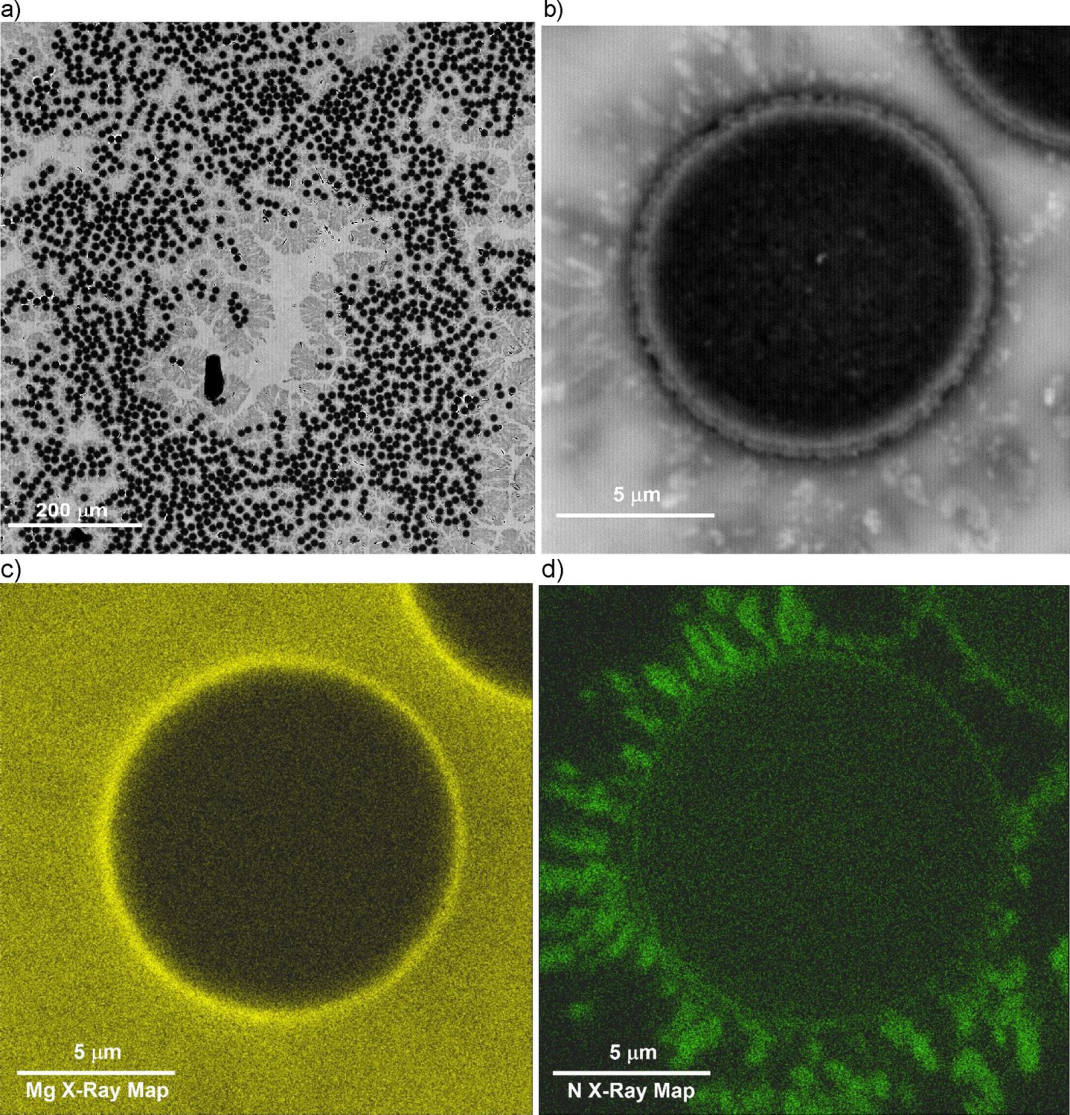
Microstructure of a fiber reinforced AMC manufactured using 3D printing technology. (a) Cross section in BSE contrast shown such that the fibers run perpendicular to the page. (b) higher magnification view used with EDS; (c) Mg characteristic X-ray map; (d) N characteristic X-ray map.
